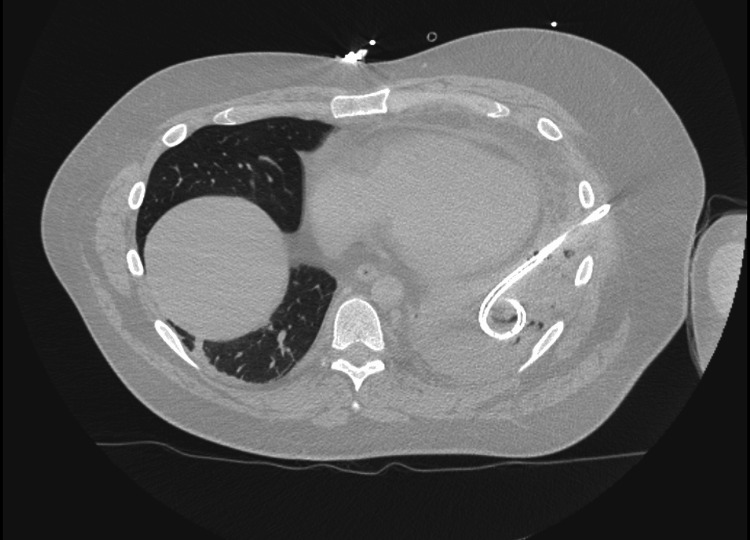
CT scan post chest tube placement
